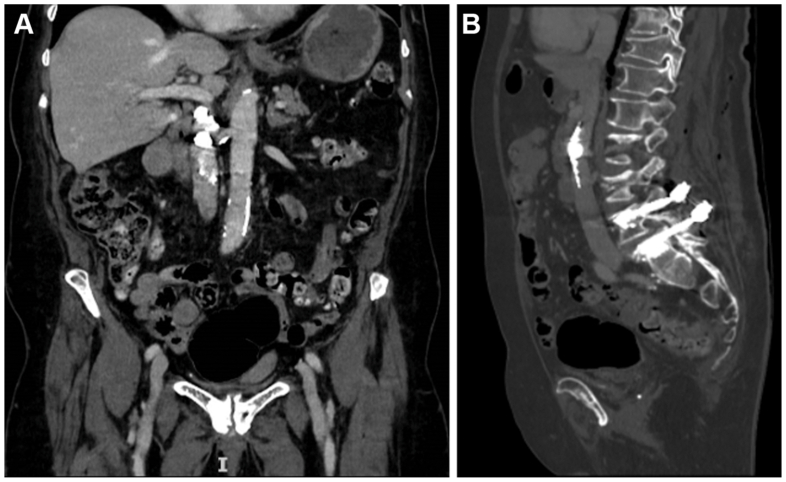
(A) Contrast-enhanced coronal computed tomography (CT) image showing a large linear cement cast partially occluding the infrahepatic inferior vena cava (IVC) at the level of the left renal vein. (B) Noncontrast sagittal CT reconstruction demonstrating the elongated cement cast extending cranially from the lumbar vertebral level.
